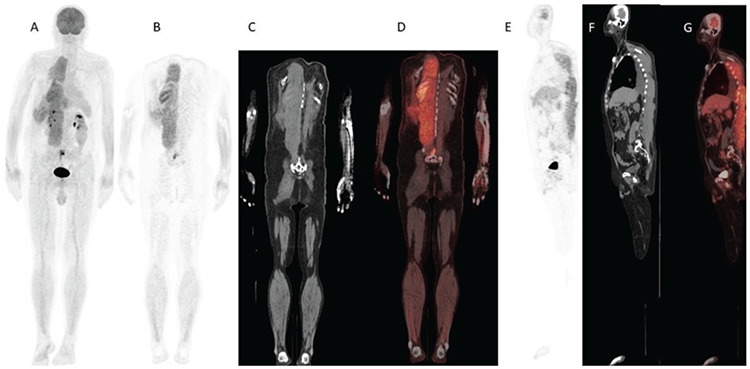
(A) Maximum intensity projection and (B) coronal PET, (C) coronal CT, (D) coronal fused and (E) sagittal PET, (F) sagittal CT, and (G) sagittal fused FDG PET/CT images demonstrate extensive and diffuse FDG uptake in paraspinal muscles. FDG: Fluorodeoxyglucose, PET/CT: positron emission tomography/computed tomography.
